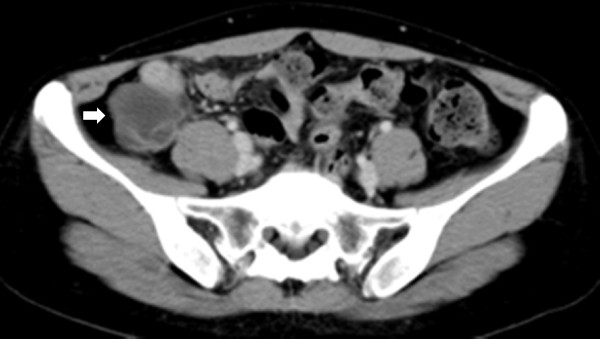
Abdominal computed tomography demonstrating a mucocele of the appendix (arrow).
